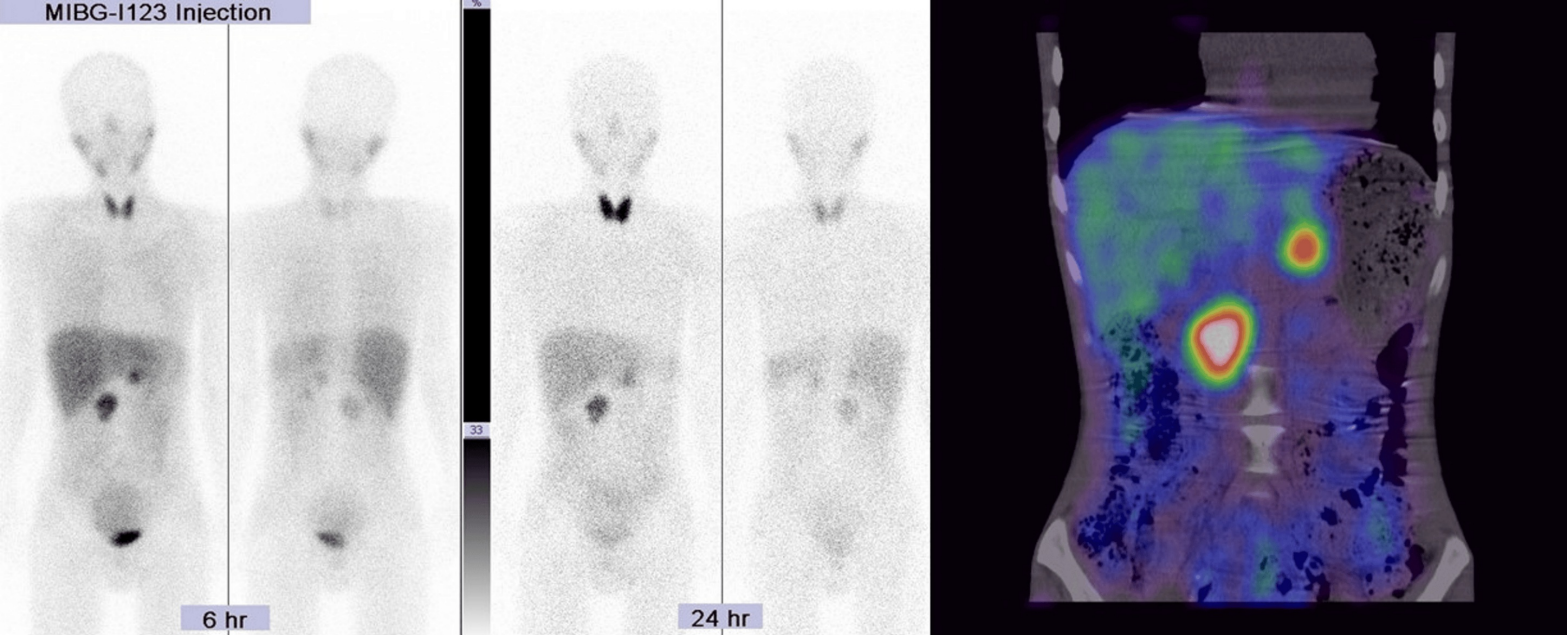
123I-MIBG scintigraphy showed uptake in the tumour
